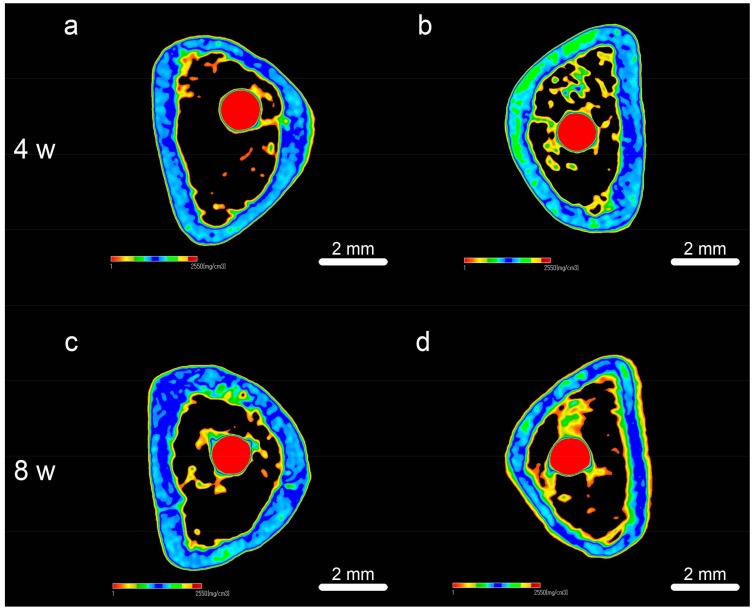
Transverse reconstructed microcomputed tomographs of (a,c) Ti and (b,d) FHA-coated implants after (a,b) 4 weeks and (c,d) 8 weeks.
